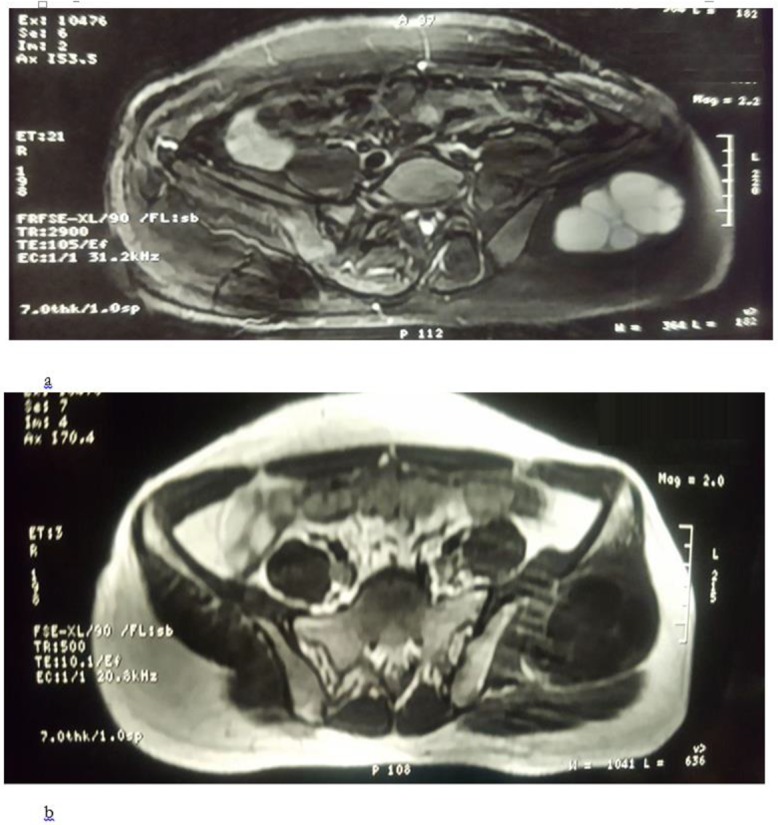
MRI showing cystic swelling in gluteus maximus in T1 (a) and T2 (b) view
